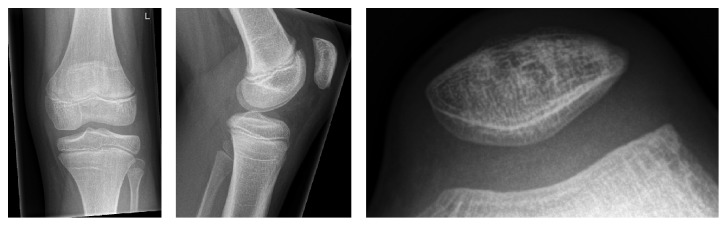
Follow-up radiographs 17 months after surgery revealing an almost normally appearing patella.
